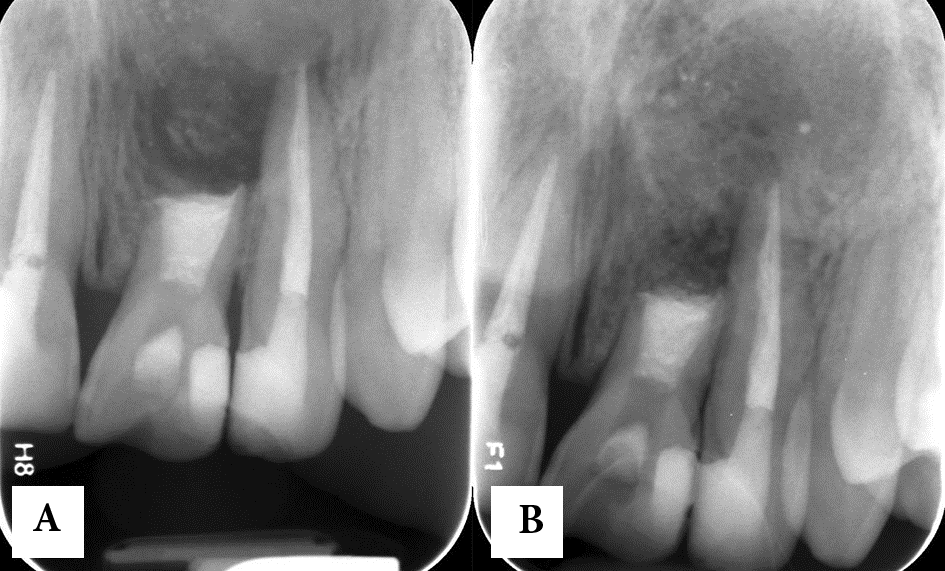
follow-up radiographs: *A) *immediately after surgery and* B) *One year later
